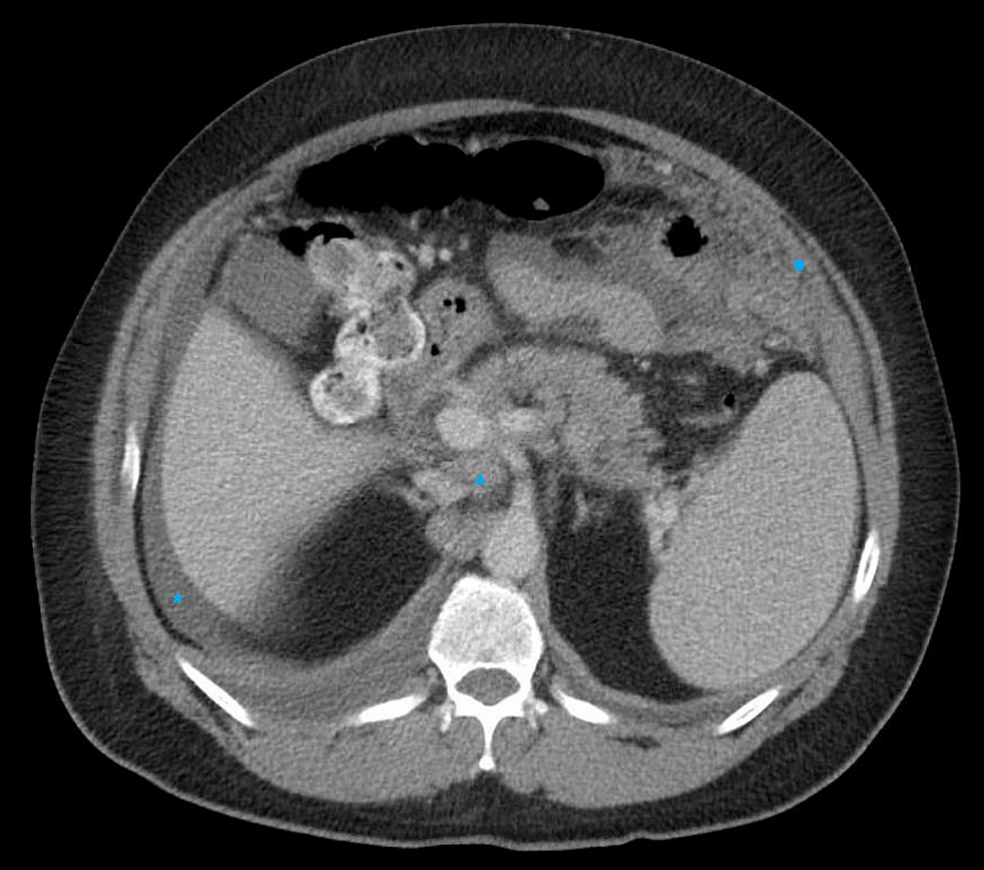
Contrast enhanced CT scan of the abdomen demonstrating bulky deposits (circle) in the left upper quadrant lateral to the transverse colon, diffuse retroperitoneal lymphadenopathy (arrowhead) seen posterior to the inferior vena cava, and mild perihepatic ascites (star).
